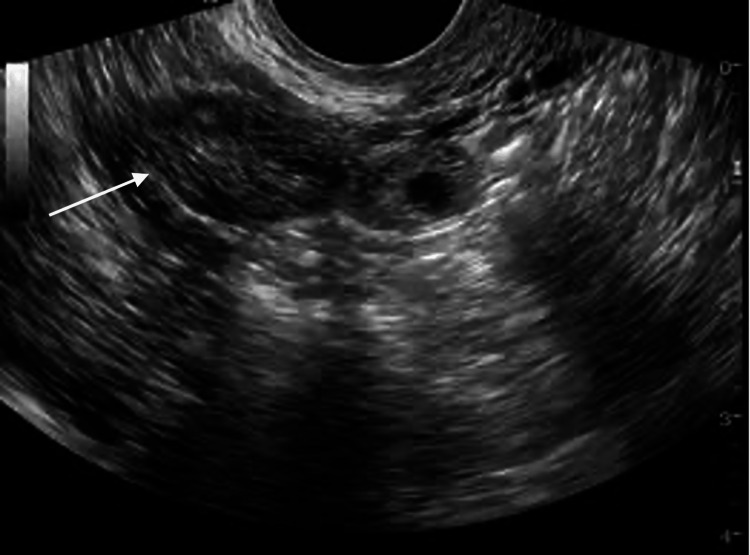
An arrow pointing to an ill-defined heterogenous large lobulated lesion measuring 9 x 4.9 x 2 centimeters in the ultrasonography of pelvis
